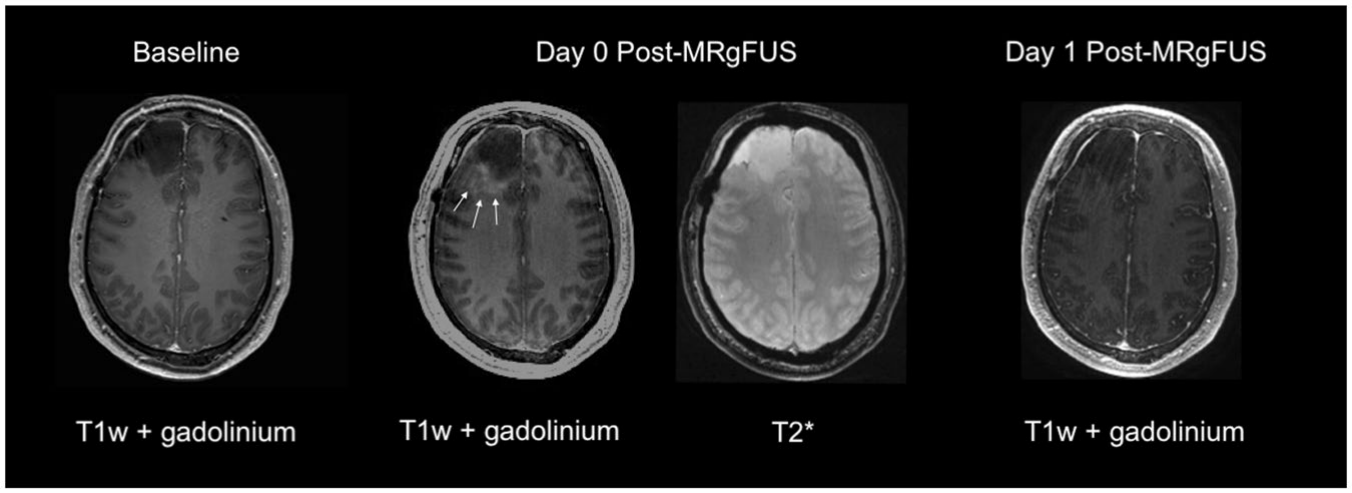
Sample T1 weighted post-gadolinium MRI from patient five obtained 30 days prior to BBB opening procedure (left), immediately following BBB disruption (middle) and 20 hours post BBB disruption (right). Ill-defined contrast enhancement is seen in the peritumoral region on images acquired immediately after MRgFUS (white arrows). This contrast enhancement has resolved in the peritumor region on the day 1 follow-up image indicating closure of the BBB. T2* sequence acquired immediately following BBB disruption for this patient show no evidence of microhemorrhages.
